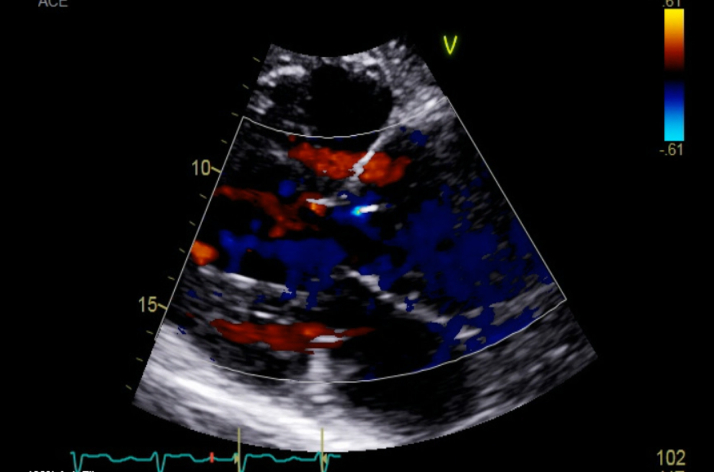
Transthoracic Echocardiogram: Parasternal Long Axis-View Color Doppler TTE demonstrating severe aortic regurgitation. Aortic valve leaflets are not well visualized. The aortic root is severely dilated measuring 6.5 cm. The ascending aorta is severely dilated measuring 6.2 cm. TTE = transthoracic echocardiography.
